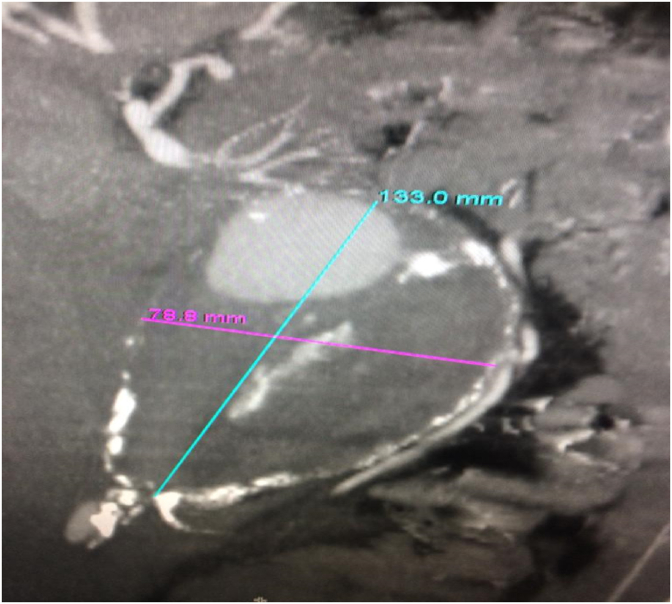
Multislice CT scan showing the dimensions of the aneurysm; 13 × 8 cm.
